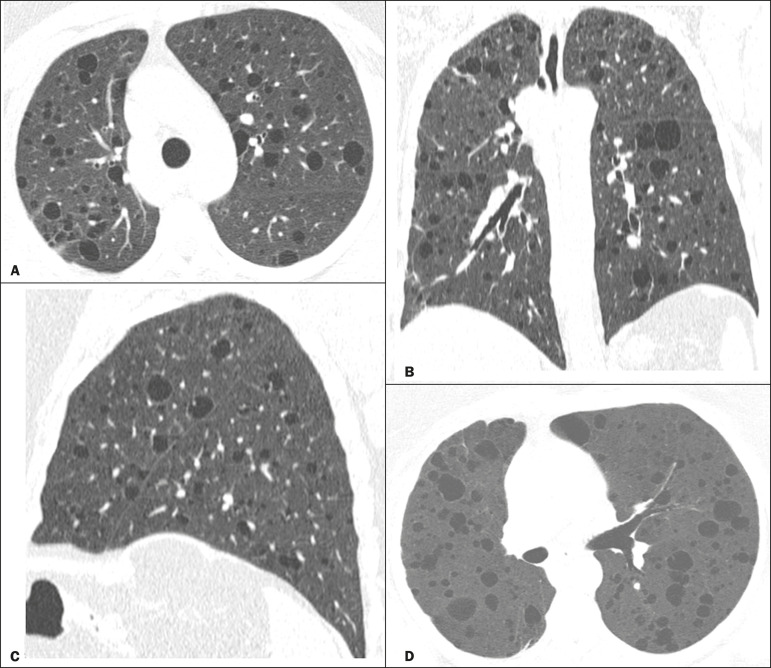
A 54-year-old woman with LIP related to Sjögren syndrome. Axial (A), coronal (B), sagittal (C), and minimal-intensity projection (D) chest CT scans show multiple bilateral pulmonary cysts with a random distribution.
